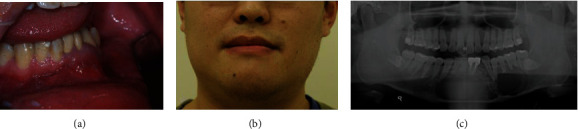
Initial findings. (a) Intraoral view shows a raised mass over the left buccal vestibular area. (b) Extraoral view shows slight facial asymmetry. (c) Panoramic radiograph reveals multilocular radiolucent lesion over the anterior and left mandible.
